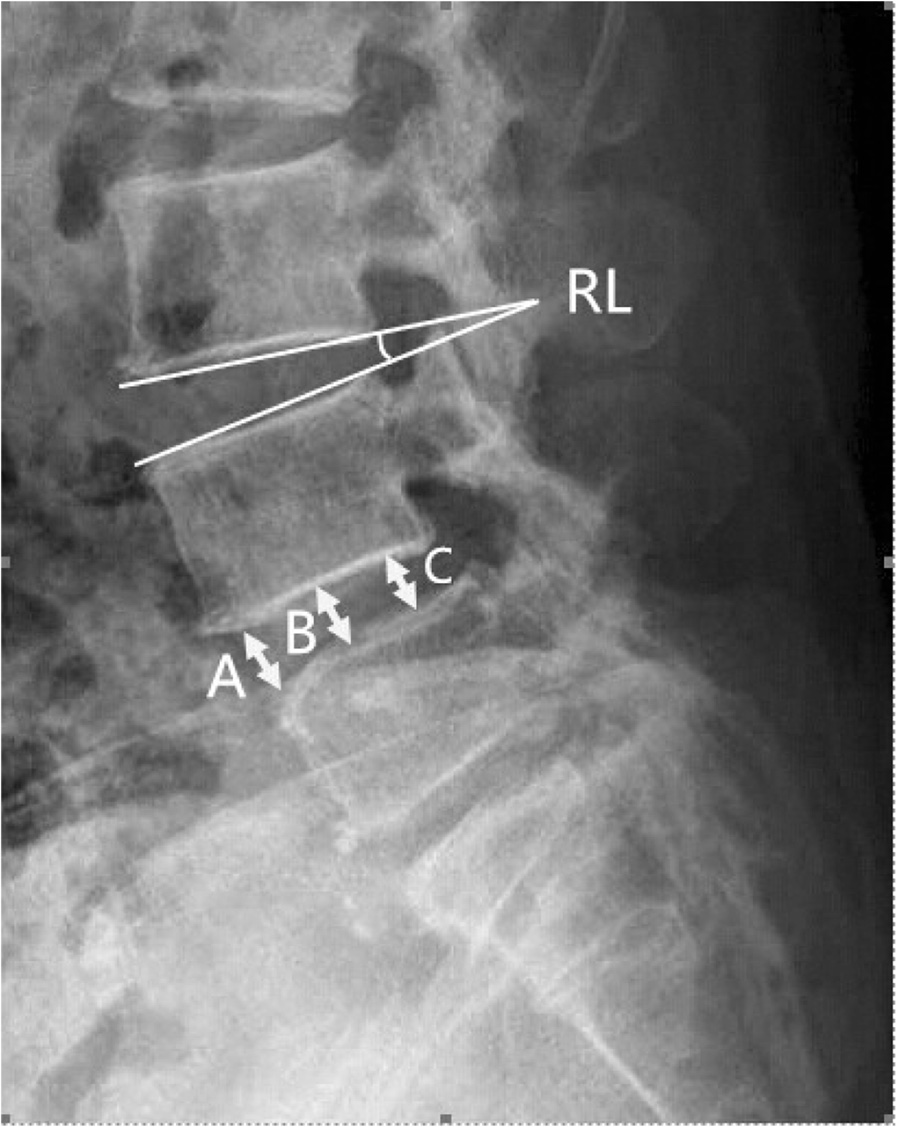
Radiographic measurements of the lumbar disc height: A anterior disc height, B middle disc height, C posterior disc height. Disc height = (A + B + C)/3 (mm). Measurement of regional lordosis (RL): the angle between the upper and lower edges of the intervertebral disc
